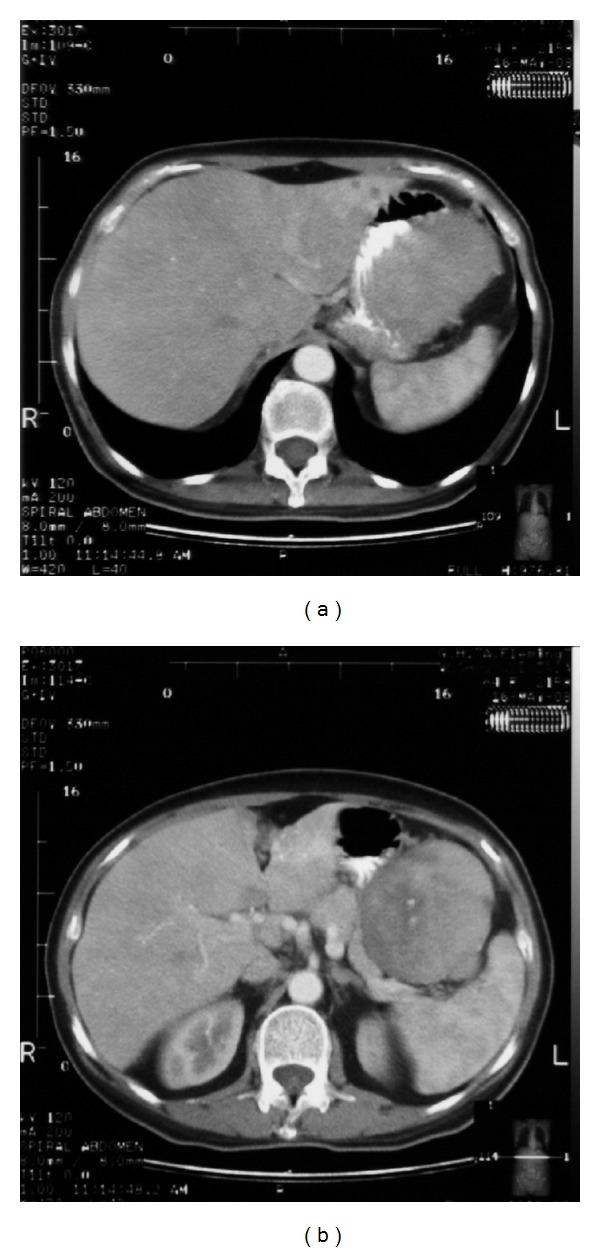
A contrast enhanced abdominal CT scan shows a large, 13.5 × 7.7 × 8.5 cm, heterogeneously enhanced mass. The mass appeared to adhere to the stomach (a). There is a clear plane between the mass and the adjacent spleen and pancreas (b).
